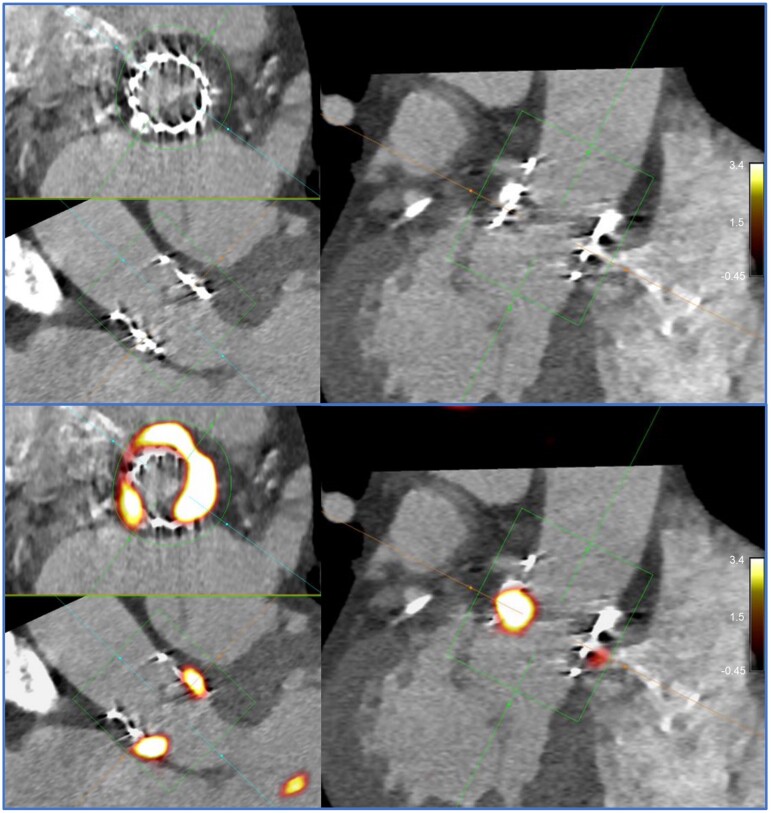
Hybrid ^18^F-NaF PET/CT acquisition in a patient with known TAVR degeneration. Intense tracer signal is seen localized to the TAVR, in particular the position of the prior non-coronary cusp of the aortic valve. In this case, TBR_max_ was calculated at 4.1 (SUV_max_ value 5.7, mean left atrial blood pool 1.4±0.1) and SNR was 47.3 after application of motion correction.
